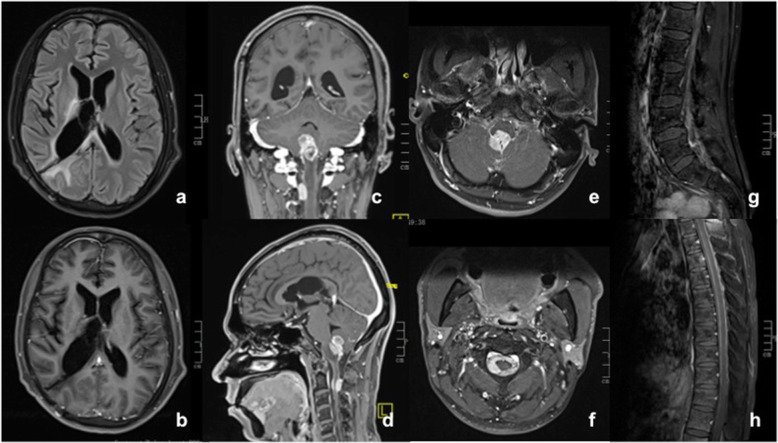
Axial a T2 FLAIR and b T1 contrast sections of brain showing near complete resolution of the primary lesion. c–f There is interval development of heterogeneously enhancing mass lesion (2.4 × 2.0 × 1.4 cm) in the inferior part of fourth ventricle causing marked compression on dorsal medulla. Another heterogeneously enhancing nodular lesion (1.1 × 0.6 × 1.4 cm) seen in right posterior subarachnoid space at the level of C2 vertebra causing mild compression of the posterior cervical spinal cord. Abnormal leptomeningeal enhancement is seen along the surface of brainstem and cerebellum. g, h Abnormal leptomeningeal enhancement is also seen along the surface of entire spinal cord with thickening and clumping of the cauda equina nerve roots
